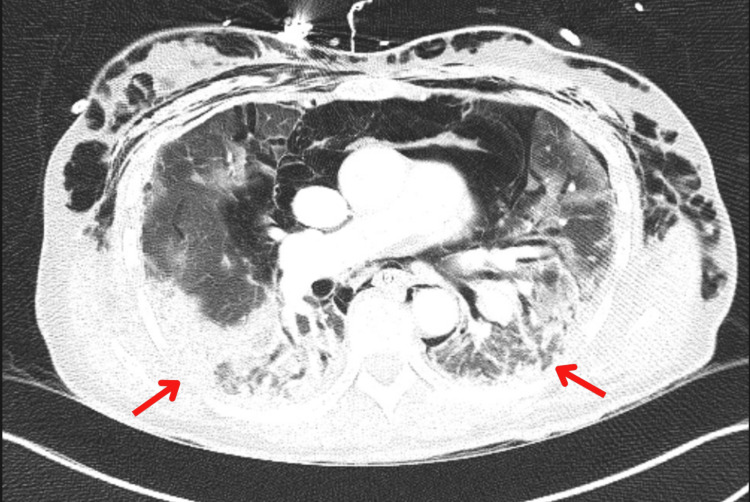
Repeat CT from a month later shows progressive interstitial lung disease (red arrows) CT: computed tomography
